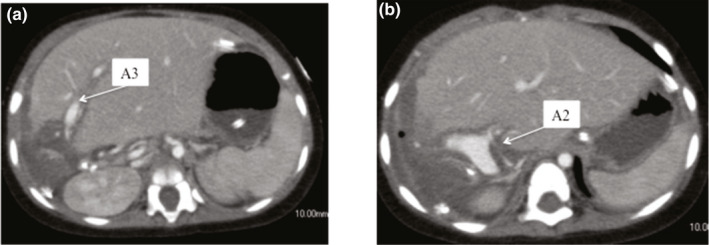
Postoperative CT images in Case 1. A, CT image of the reconstructed hepatic artery (A3) on postoperative day 7. B, CT image of the reconstructed hepatic artery (A2) on postoperative day 7
